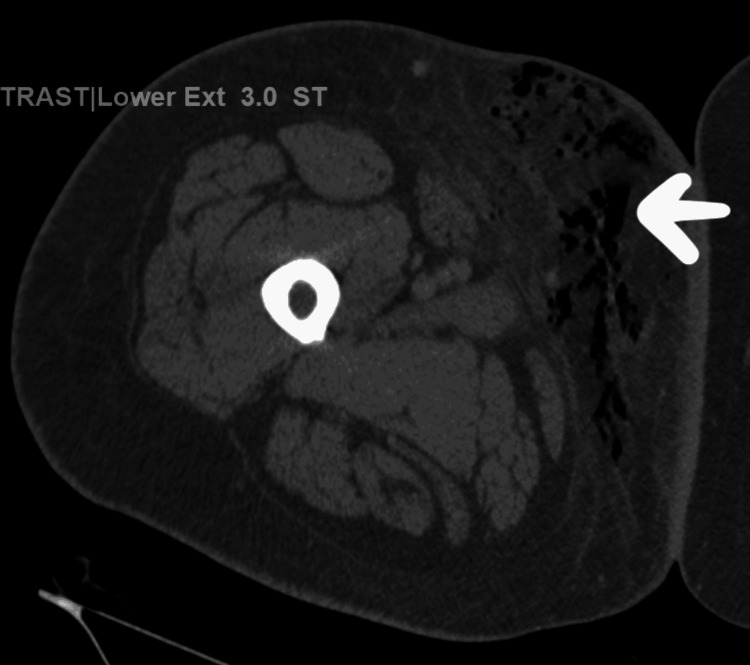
Computed tomography (CT) of the right lower extremity revealed punctate bubbles of gas in the subcutaneous tissue in the pelvis which extended to the medial right thigh consistent with NF ( arrow-marked area on the image)
